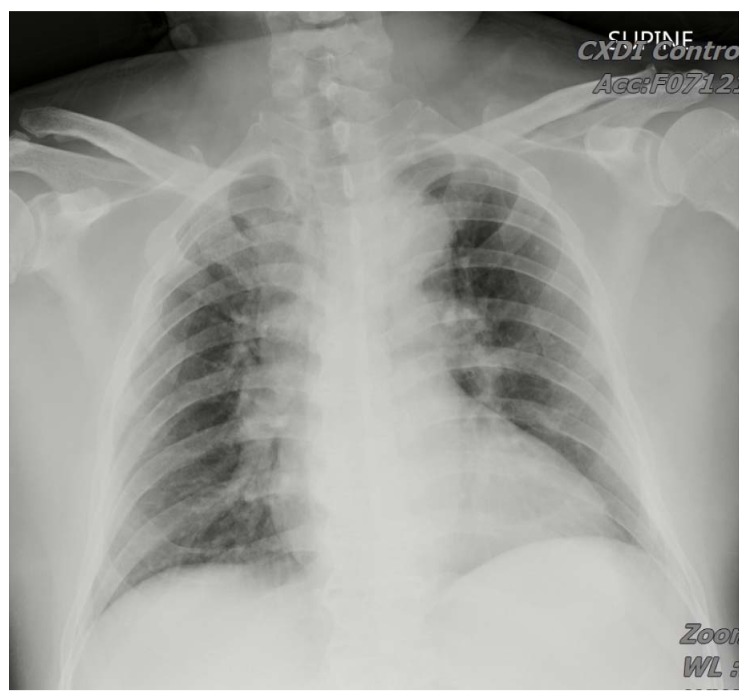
The chest X-ray revealed bilateral diffuse infiltration.
